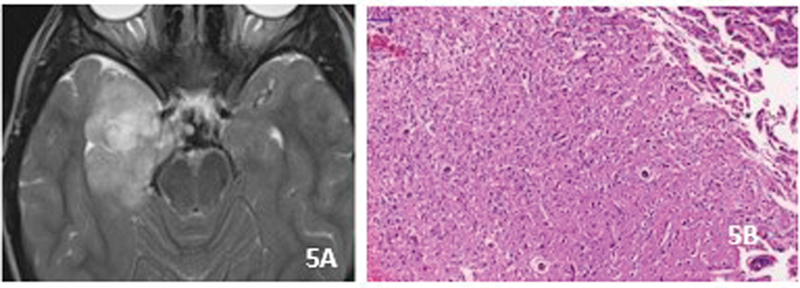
PLNTY. A) Axial FLAIR. Heterogeneity of hyperintense area. No mass effect or oedema. B) Diffuse proliferation of oligodendroglial-like cells.
